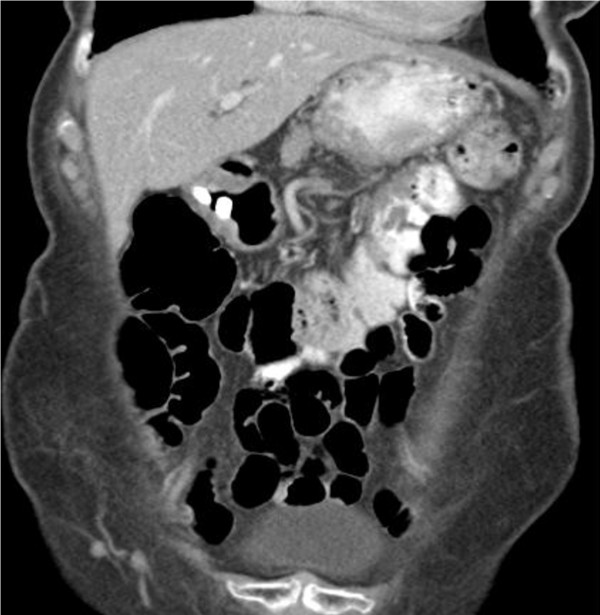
Coronal CT scan post-gastroscopy revealing gastro-colic fistula demonstrated by oral contrast in the stomach and distal transverse colon and absence of contrast in the duodenum. There are associated inflammatory changes around the transverse colon.
